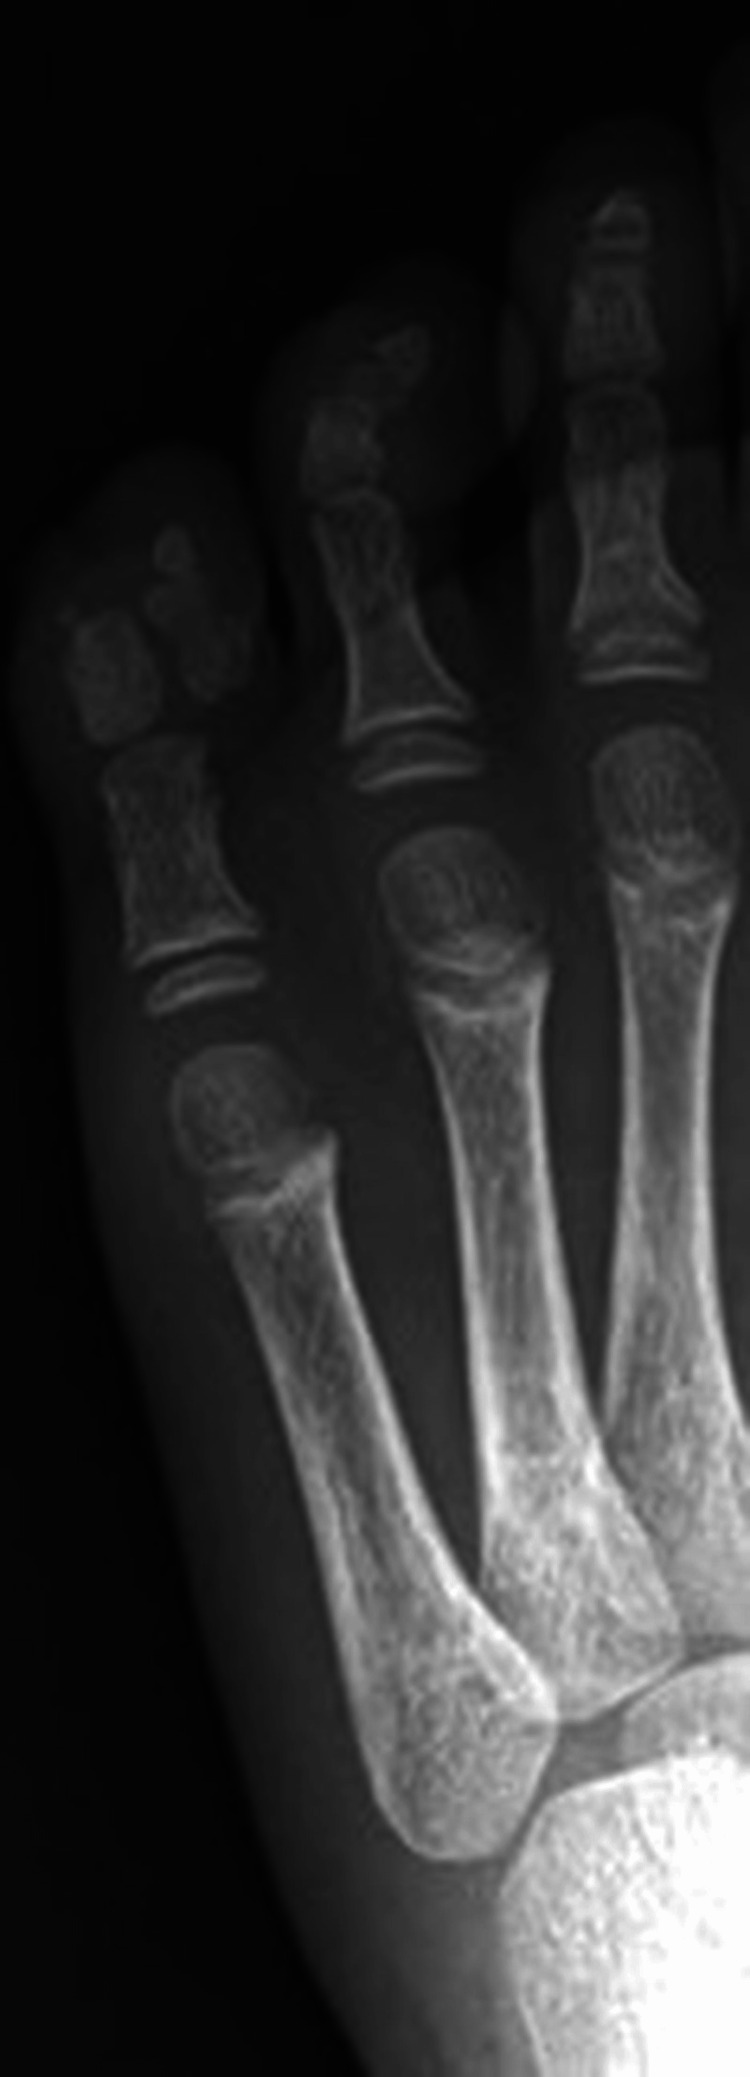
Preoperative radiograph of the left foot demonstrating polysyndactyly of the third, fourth, and fifth toes (right to left) Observed on the left foot is polysyndactyly of the third, fourth, and fifth (right to left) toes with medial deviation and duplication of the fifth toe (extreme left), which was selected for excision.
